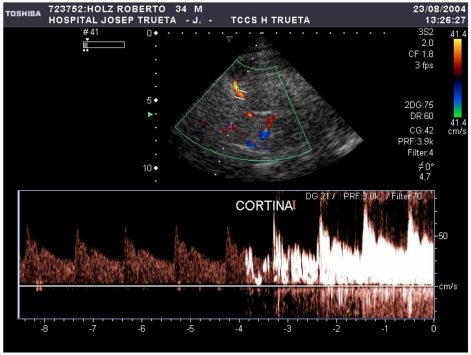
Massive RLSh by transcranial colour coded duplex sonography detected 3 seconds after contrast infusion (agitated saline solution, 9 mL of saline solution and 1 mL of air). Video can be downloaded at http://www.telefonica.net/web2/jserenal/pulmonaryshunt/fistula.htm
